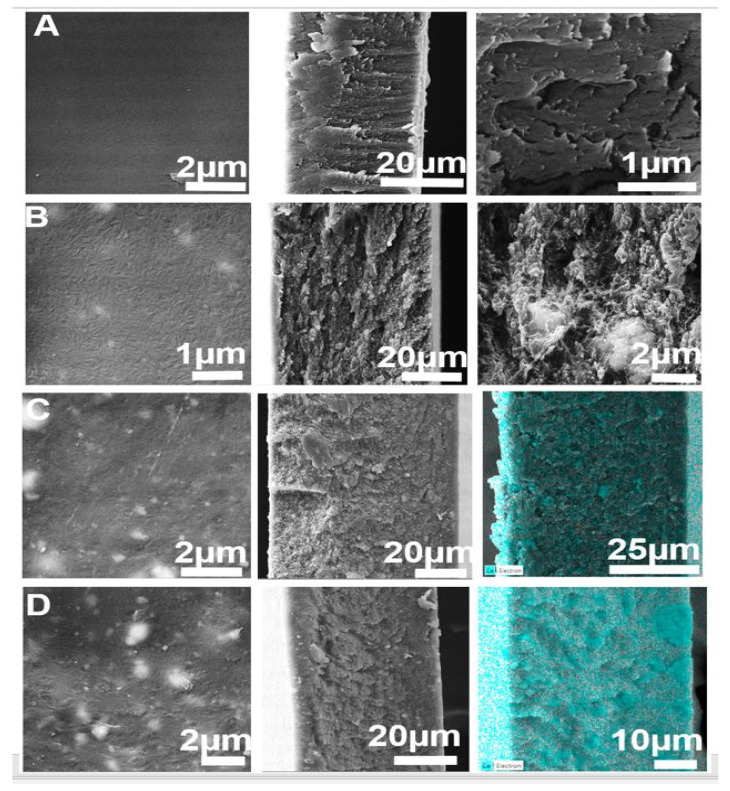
SEM images of the surface, cross-section, the magnified portion of the cross-section and EDS overlaid images: (A) CTA, (B) CTA-CeO_2_ (0.32), (C) CTA-CeO_2_ (0.64) and (D) CTA-CeO_2_ (0.9), across the rows, respectively.
